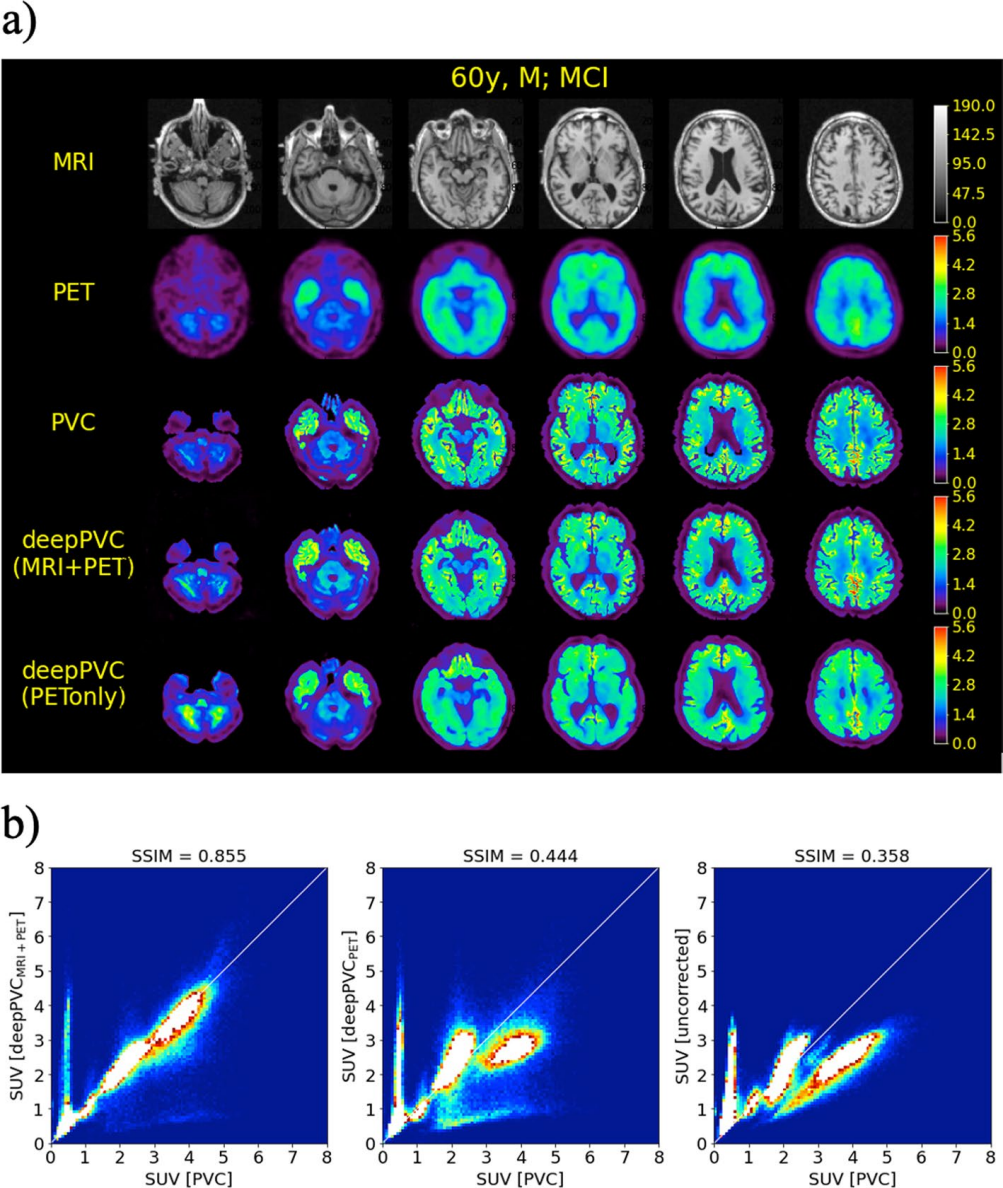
MR images, SUV maps (a), and 2D histograms of the PV-corrected map (b) for the representative case of PiB-positive (60 years old; male; MCI). The 2D histograms, left to right, represent maps predicted with deepPVC_MRI+PET_, deepPVC_PET_, and uncorrected PET, respectively. The white lines on the histograms indicate perfect correspondence with the real PV-corrected SUV. MCI, mild cognitive impairment; MR, magnetic resonance; PVC, partial volume correction; SUV, standardized uptake value
